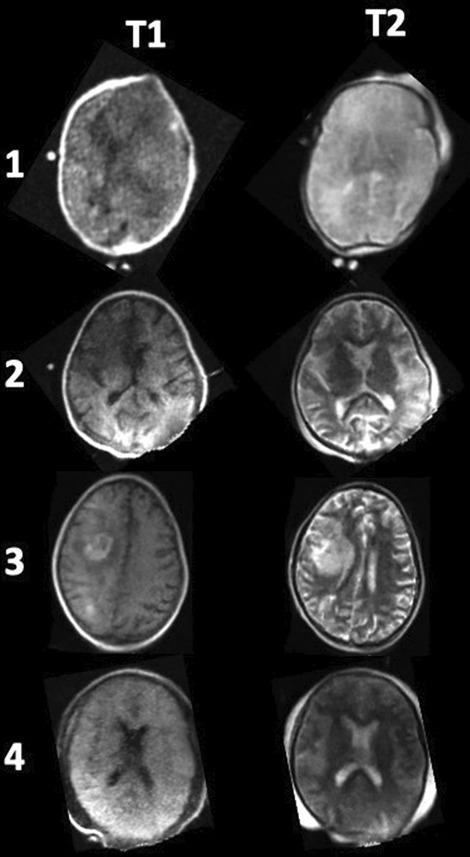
Representing T1 and T2-weighted images from the four patients receiving pMRI during ECLS treatment. In Patient 3 pMRI showed right hemispheric intracerebral hemorrhage. All other patients did not have signs of hemorrhage or stroke. Acquisition times: T1 (5m35s) and T2 (5m50s)
